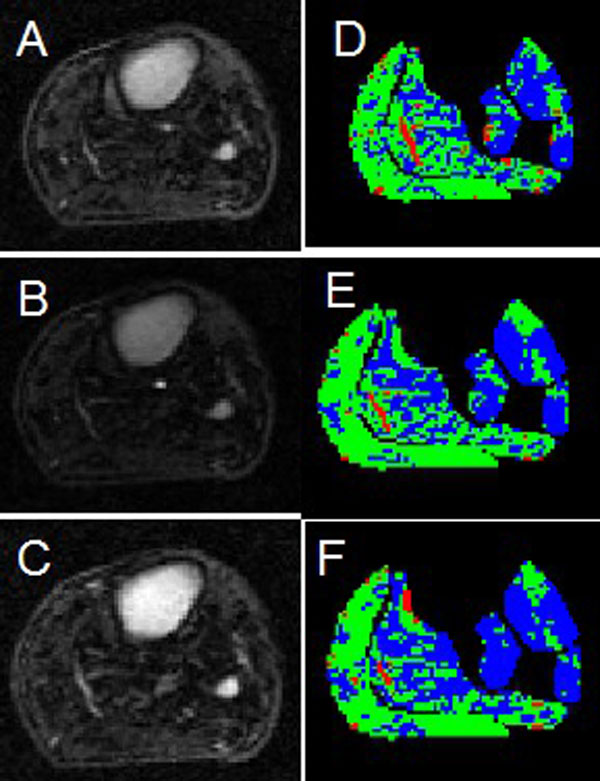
CE-MRI images from a PAD patient (panels A-C) prior to contrast arrival (CA, panel A), at peak arterial contrast enhancement (panel B), and 2 minutes post CA. Panels D-F show the respective perfusion maps where blue, green, and red colors indicate hypointense, isointense, and hyperintense regions, respectively.
